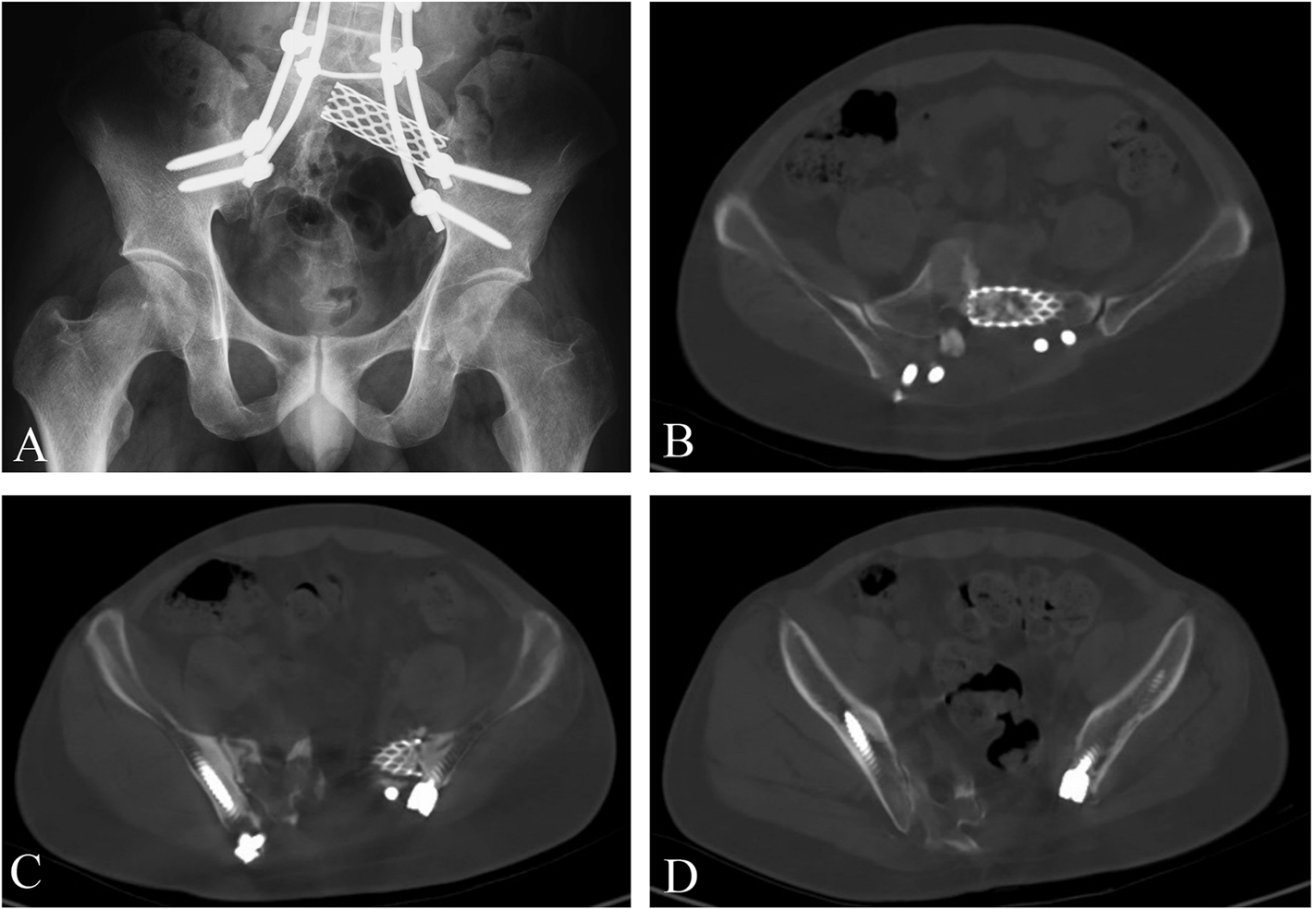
A-D. The follow-up at one and half year of patient no. 2. The patient can walk independently with mild gait abnormal. Pelvic x-ray were shown. The transverse CT scan (B, C and D) of different levels showed bony cut at both sacrum and ilium
